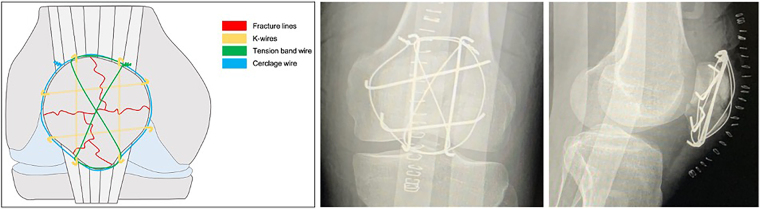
The tension band technique combined with circumferential cerclage wiring. Postoperative radiographic images of the patient showing final fixation of the severe comminuted patellar fracture.
